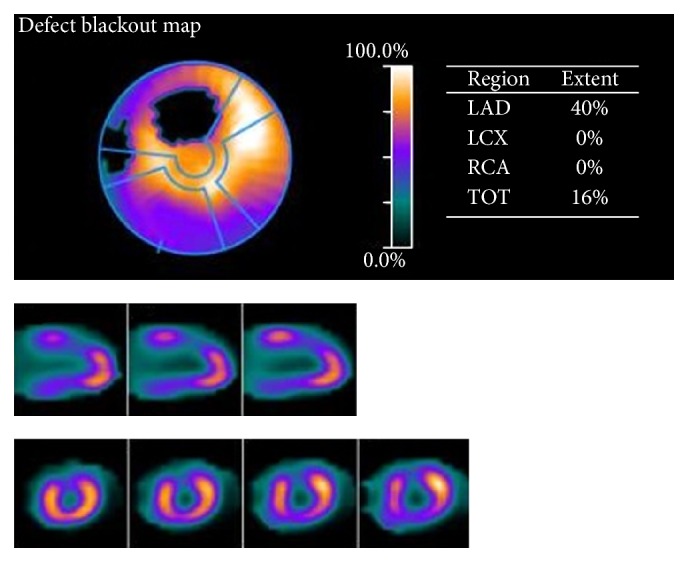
PET assessment. Comment: 59-year-old male with known ischaemic heart disease (requiring bypass grafting) presents for PET assessment in the context of new-onset angina. PET assessment findings of scintigraphic evidence of a reversible perfusion defect of the mid third of the anterior wall is noted. This gated data suggests a high-grade stenosis supplying this region. Noted normal left ventricular systolic function at rest with an inducible wall motion abnormality and significant fall in LVEF with pharmacological stress.
